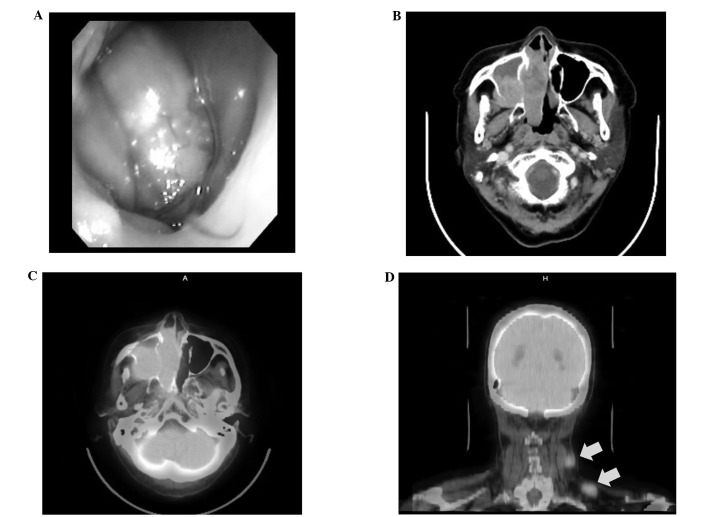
(A) Nasal endoscopic view of an occupying mass in the right nasal cavity. (B) Computed tomography scan revealing the destructive lesion occupying the right maxillary sinus and right nasal cavity with loss of posterior boney walls of the antrum. Fluorodeoxyglucose positron emission tomography revealed high accumulation in (C) the primary tumor and (D) left cervical lymph nodes (arrows).
